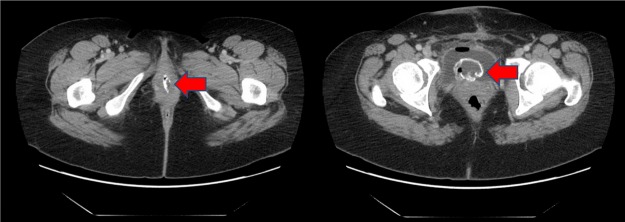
CT abdomen and pelvis axial section. High attenuation lesion in the urethra and bladder lumen (*arrow*).
